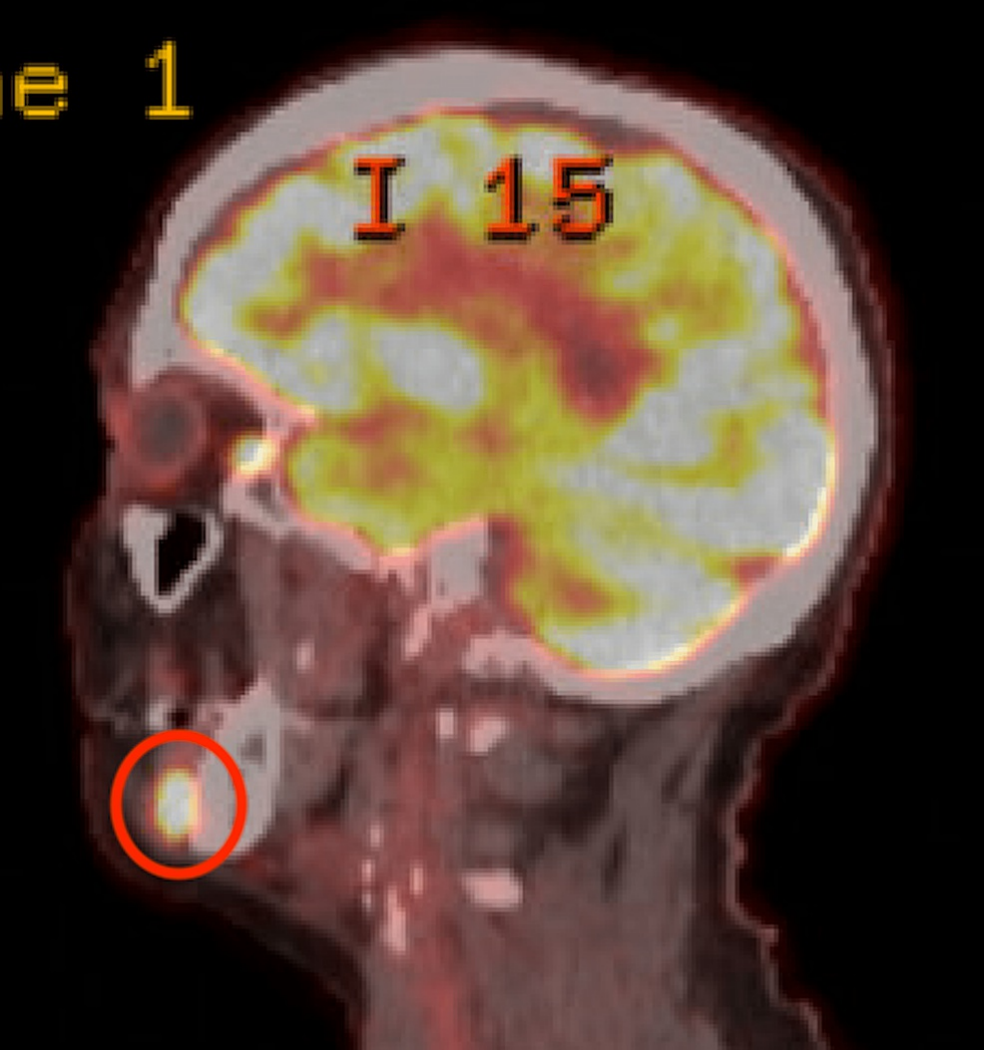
PET-CT sagittal view showing level Ib hypermetabolic lymph node. PET-CT: positron emission tomography-computed tomography
